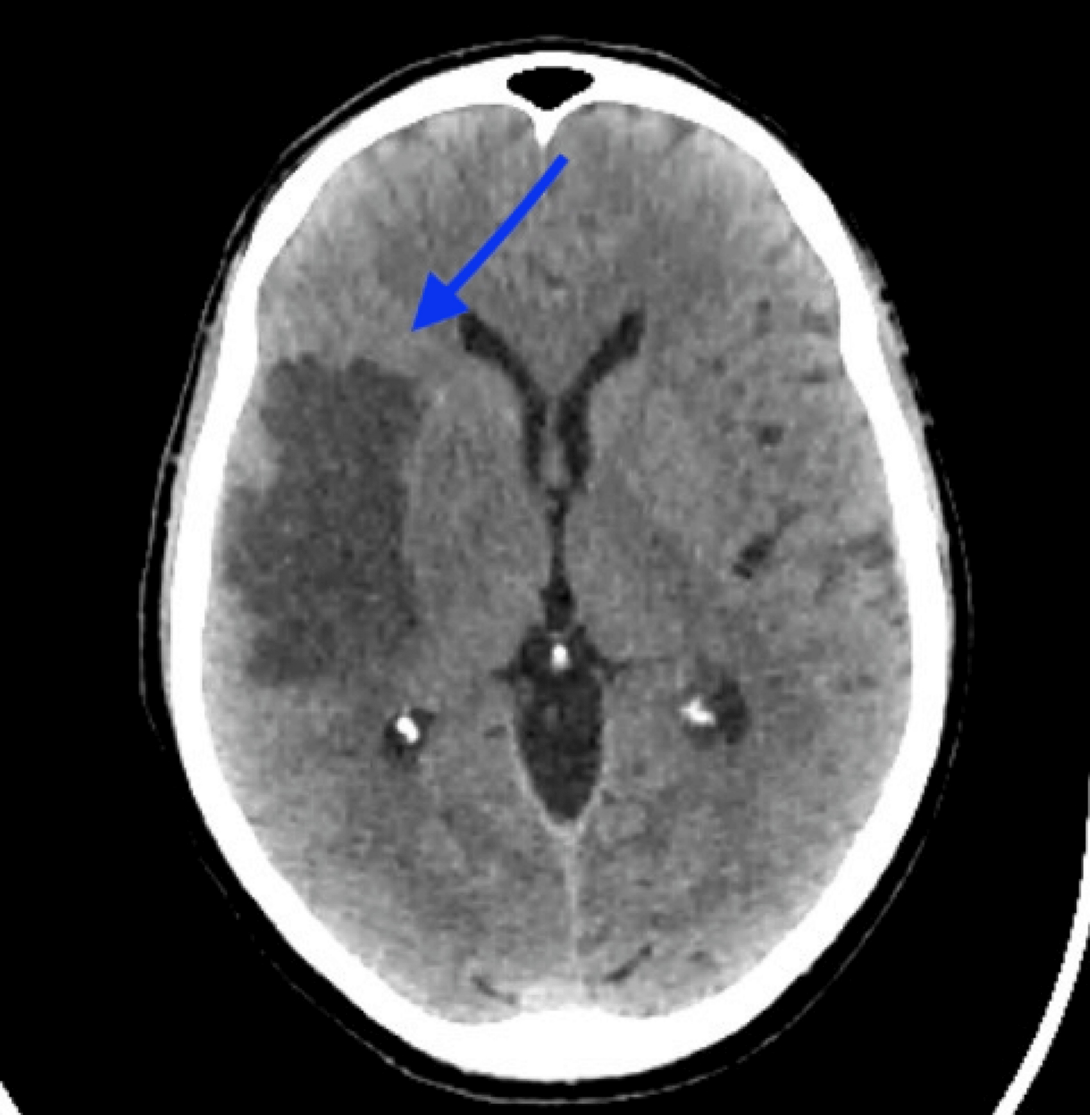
Brain CT scan on admission. Brain CT demonstrating a large right middle cerebral artery territory infarction, with significant hypodensity and loss of grey-white matter differentiation ( blue arrow). These findings are consistent with an acute ischemic stroke involving the right middle cerebral artery territory.
